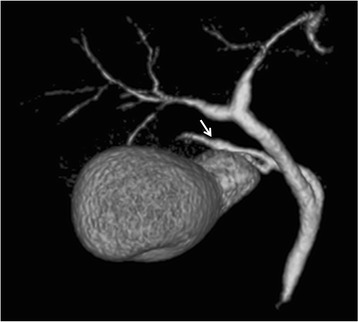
Three-dimensional DIC-CT image (ventral view) showing a type IV accessory hepatic duct. The accessory hepatic duct drained the right posterior section (*arrow*) and was classified as belonging to the posterior branch type
